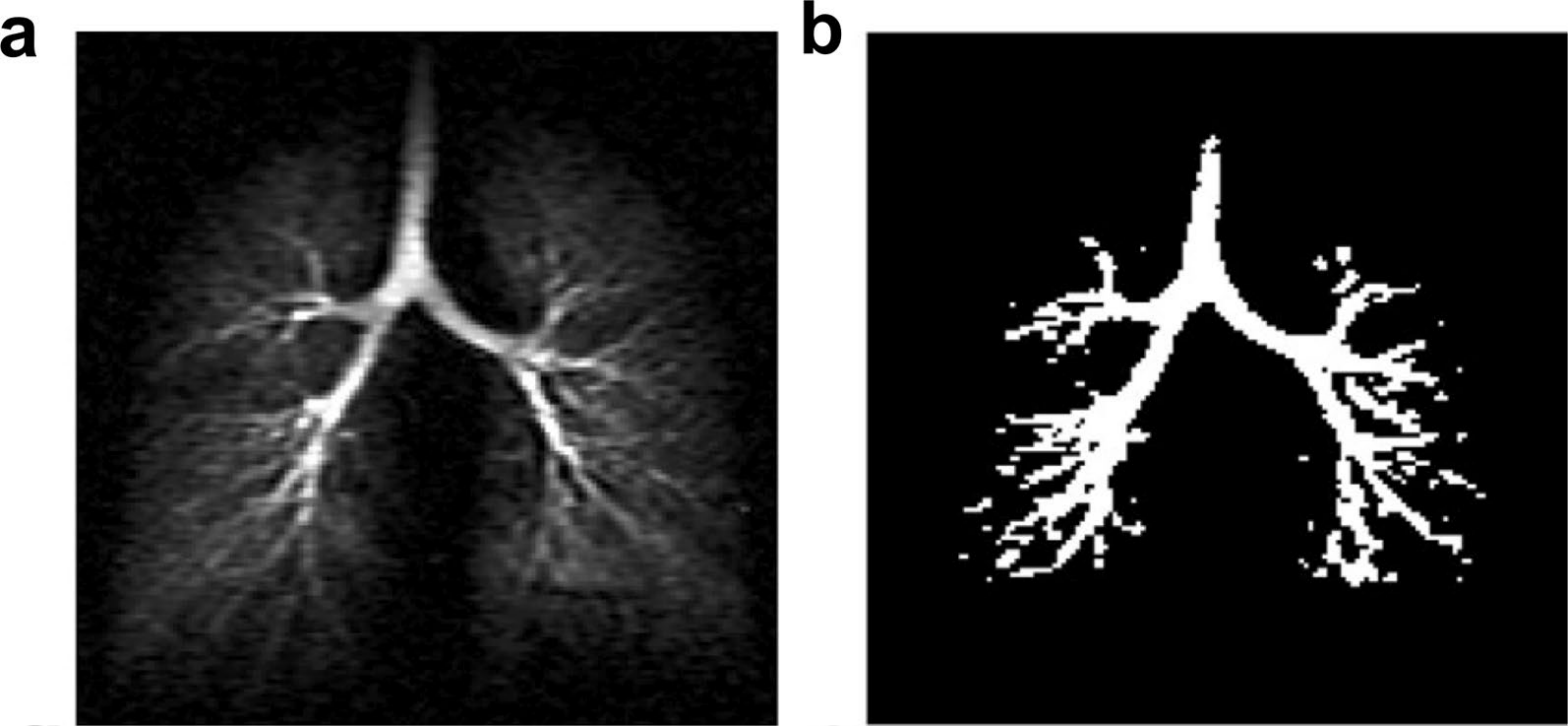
Representative dynamic coronal MR images using hyperpolarized 3He MRI. Thresholding a dynamic projection image. a Before thresholding; b After thresholding. Reproduced with permission from John Wiley and Sons (License Number: 532430065345). Reproduced with permission from John Wiley and Sons (License Number: 5324291003853). NMR Biomed. 2013 April; 26(4): 424–435
